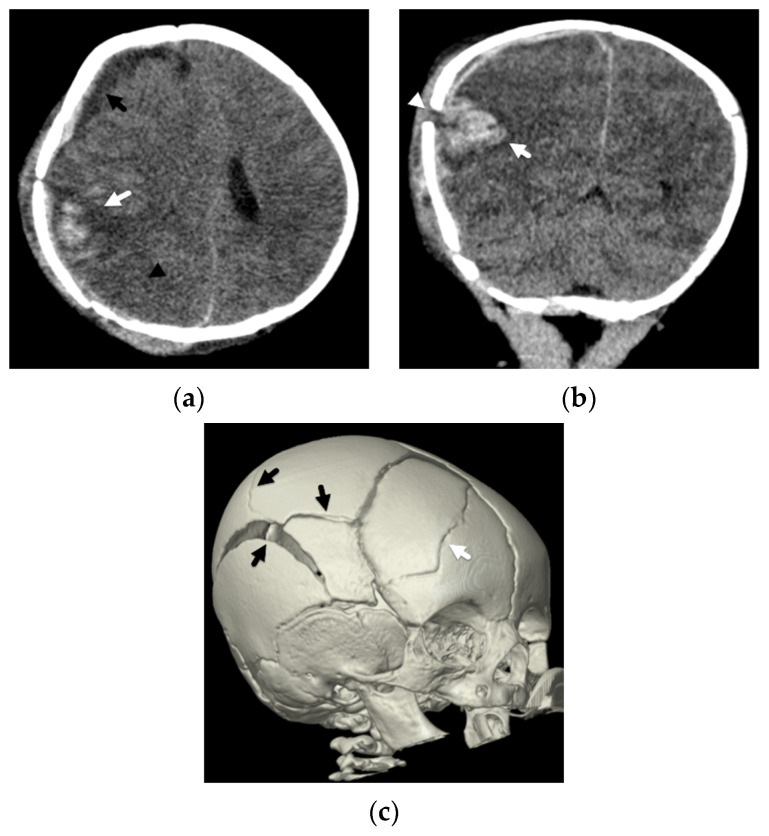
Skull fracture and hemorrhagic contusion in a 2-month-old female presenting with the history of a fall off a couch. (a) Initial axial CT demonstrates a hemorrhagic contusion at the posterior temporal-parietal lobe junction (white arrow) with mixed but predominantly low-attenuation hemorrhagic subdural (black arrow). More focal loss of gray-white matter differentiation (black arrowhead) may also suggest coexistent venous infarction or bland contusion; (b) coronal CT reconstruction shows the hemorrhagic contusion (white arrow) deep to a diastatic right parietal bone fracture (white arrowhead); (c) three-dimensional reconstruction of the skull demonstrates a mildly complex right parietal bones fracture (black arrows) and right frontal bone fracture (white arrow).
